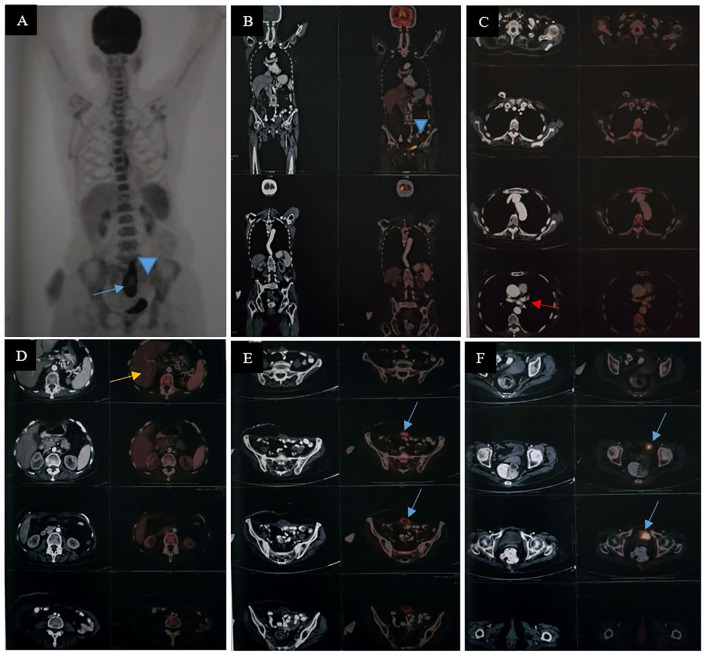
Whole body PET/CT, maximum intensity projection (MIP) (17 October 2024) demonstrated no abnormal FDG uptake in the anastomosis site [(A, B) marked by a blue arrowhead]. There was a marginal FDG uptake area (SUV max: 6.2) with non-avid central component noted in the anterior lower abdominal wall (7 × 4.1 × 9.8 cm) in the midline following the incision line, likely inflammatory collection [(A, E, F) marked by a blue arrow]. Benign left lung nodule [(C) marked by red arrow] with mild hepatomegaly noted [(D) marked by an orange arrow].
